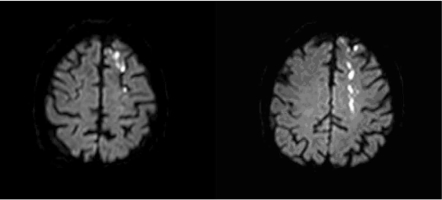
MRI-DWI showing a watershed infarct between left MCA-ACA territories.
